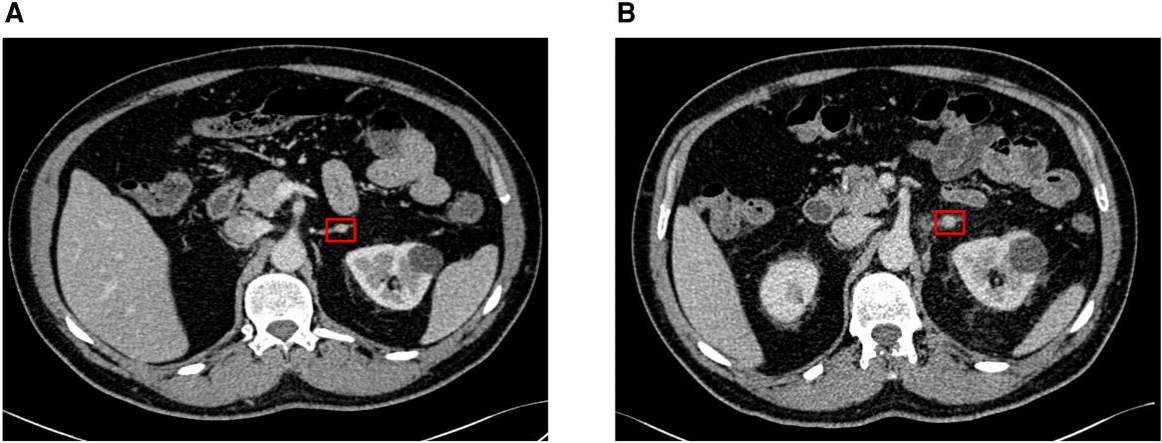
CT adrenal imaging at baseline and in the postoperative period demonstrating a left adrenal adenoma. (A) Baseline imaging demonstrating a 9-mm diameter nodule within the lateral limb of the left adrenal gland. The lesion demonstrated enhancement in keeping with a benign adrenal adenoma (absolute washout 65% and relative washout 55%). Normal configuration of the right adrenal gland. (B) Postoperative imaging demonstrating a partial left adrenalectomy and a residual 6-mm nodule within the lateral limb of the adrenal gland. Boxes in both panels depict left adrenal nodule.
